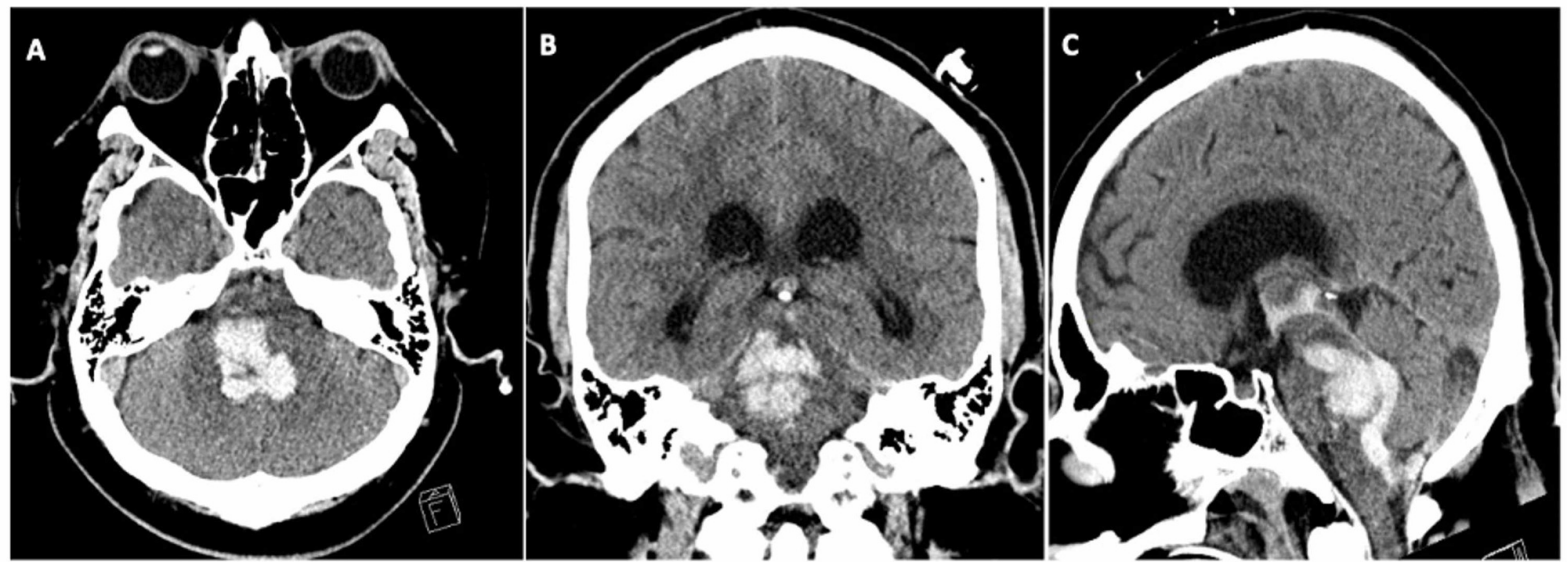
CT head without contrast revealed extensive pontine and midbrain hemorrhage with intraventricular extension involving the third and fourth ventricles and early hydrocephalus (A) Axial image at the level of the pons (B) Coronal image centered at largest diameter of intraparenchymal hematoma (C) Sagittal image showing hemorrhage extending to 4th and 3rd ventricles
